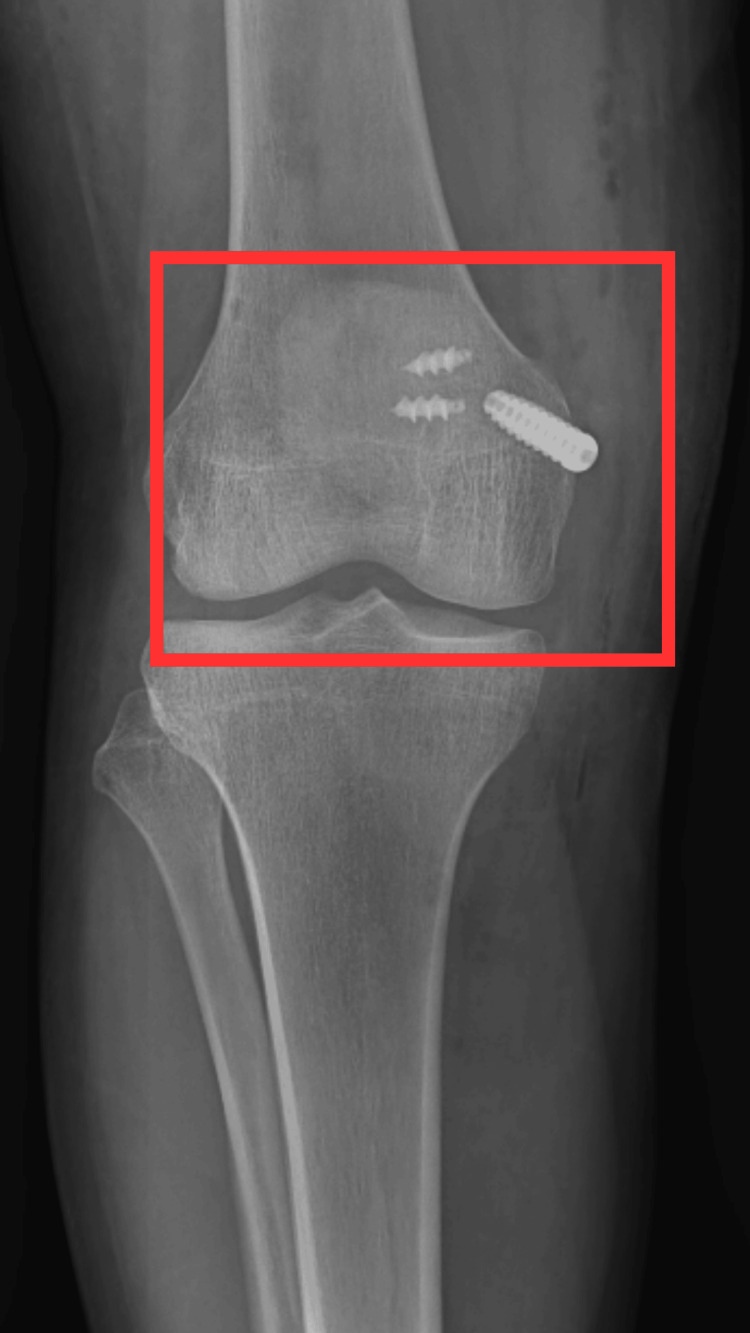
Post-operative x-ray of right knee joint
